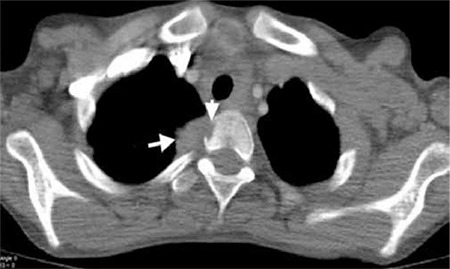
Axial CT images at bone window level demonstrate low attenuated right paravertebral soft tissue mass (arrows). Soft tissue mass invades body of the T2 vertebra and forms a low attenuated lesion in the vertebral body (arrowheads)
